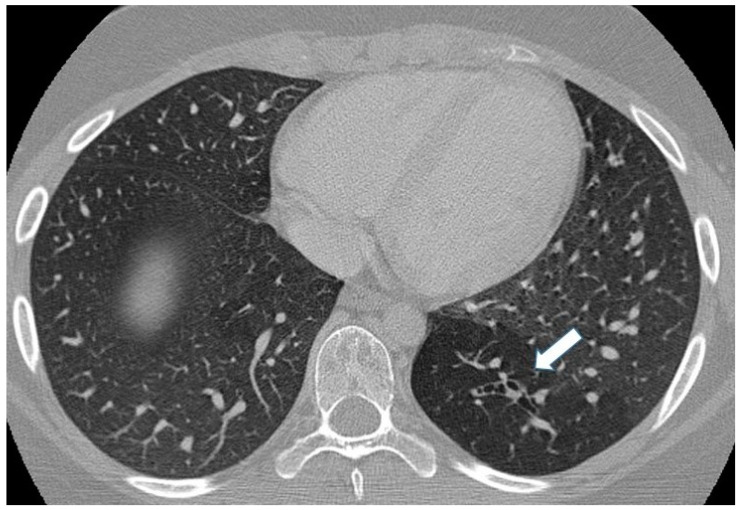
Incidental finding of bronchial atresia during a CT scan in a 15-year-old girl with lymphoma. In the left lower lobe, a tubular-shaped opacity (mucoid-filled bronchus) is associated with segmental hypoattenuation (arrow).
